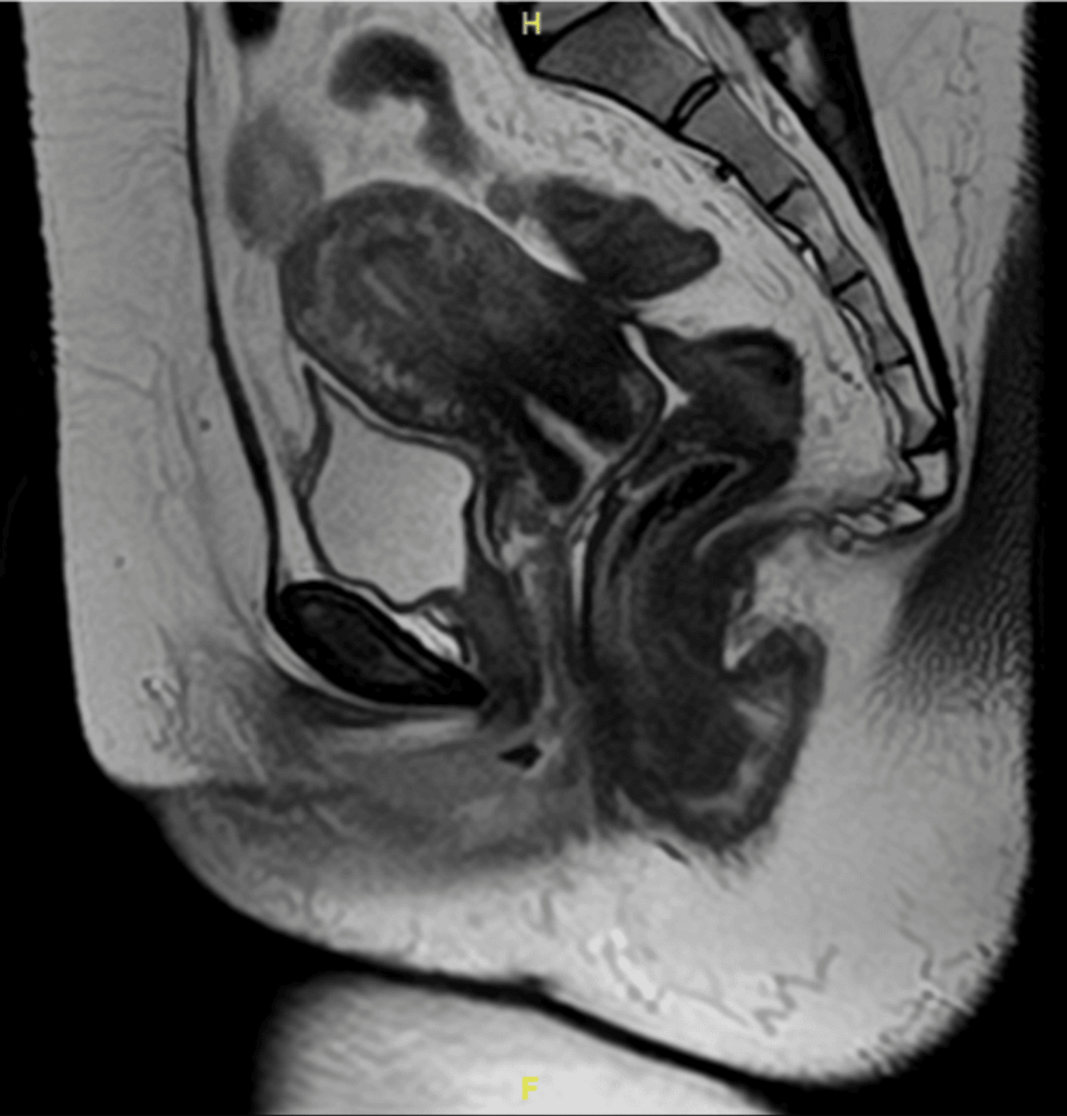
Sagittal magnetic resonance imaging of the pelvis performed six months after the completion of hormonal therapy.
